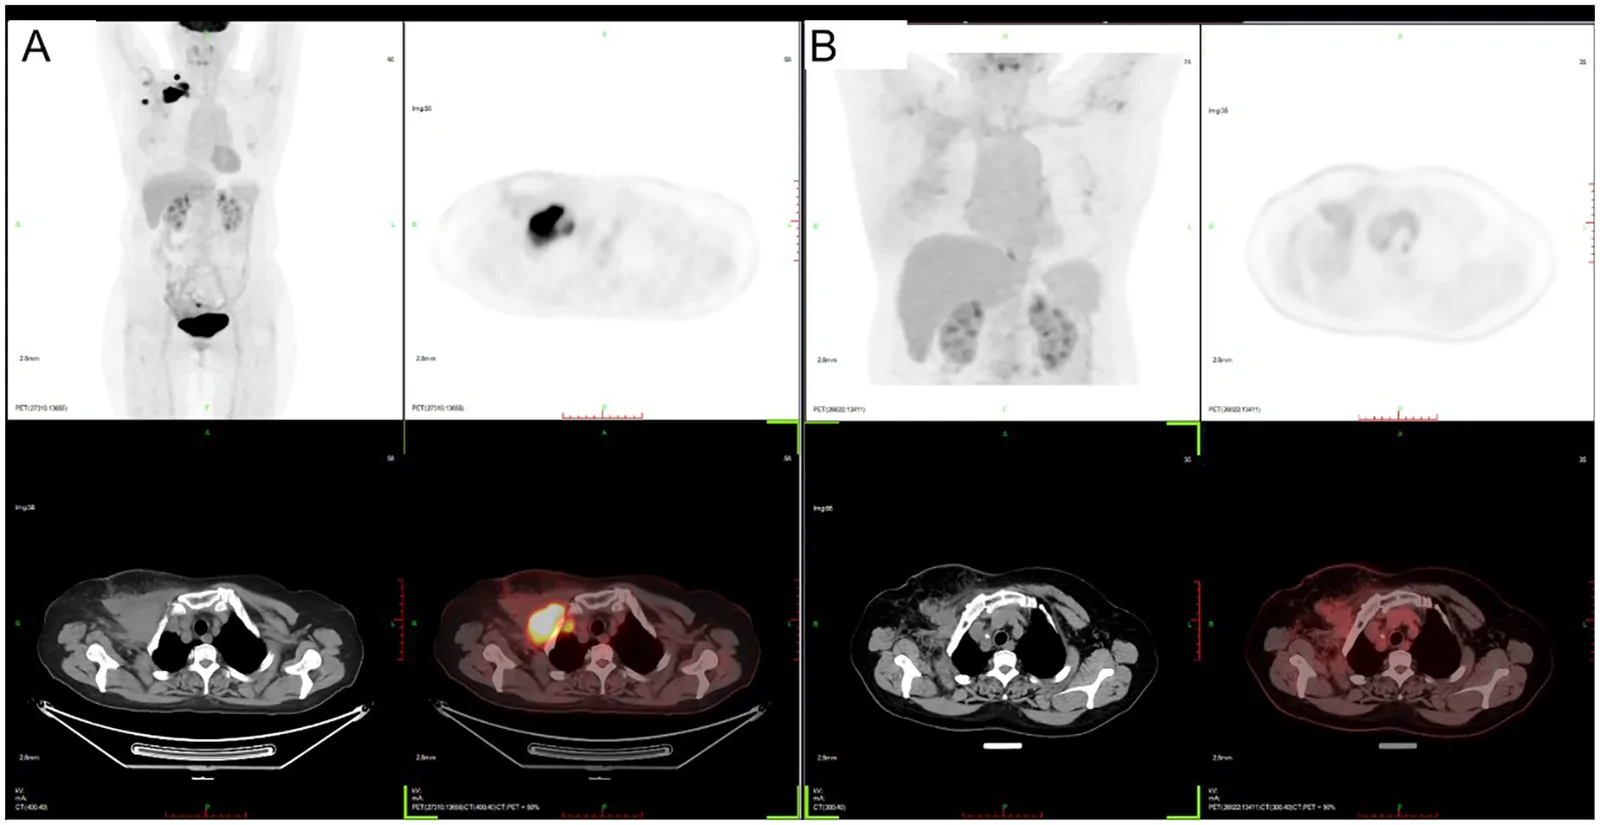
Post-treatment follow-up PET-CT (January 15, 2026): (A) were before treatment, (B) were PET/CT scan performed on January 15, 2026 (the last follow-up images). Complete metabolic resolution of previous enlarged lymph nodes in right axillary and cervical regions. No residual hypermetabolic tumor lesions, meeting RECIST 1.1 criteria for complete response (CR).
